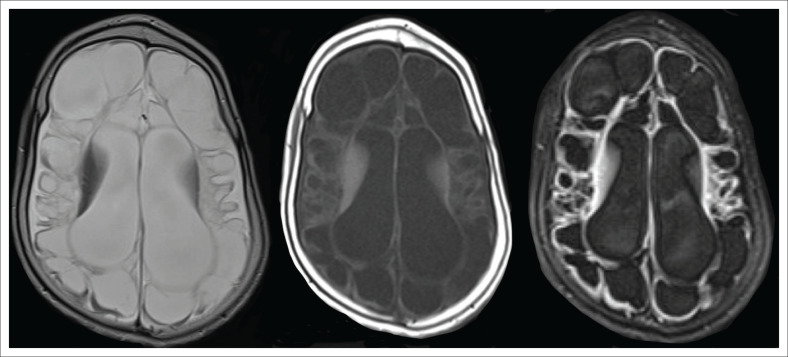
A 3-year-old male child with spastic cerebral palsy and blindness. Recorded neonatal encephalopathy with low Apgar scores and neonatal seizures. Axial T2-weighted, T1-weighted and FLAIR sequence images show extensive cerebral destruction with grey and white matter injury, cystic encephalomalacia and ex vacuo dilatation of the lateral ventricles.
